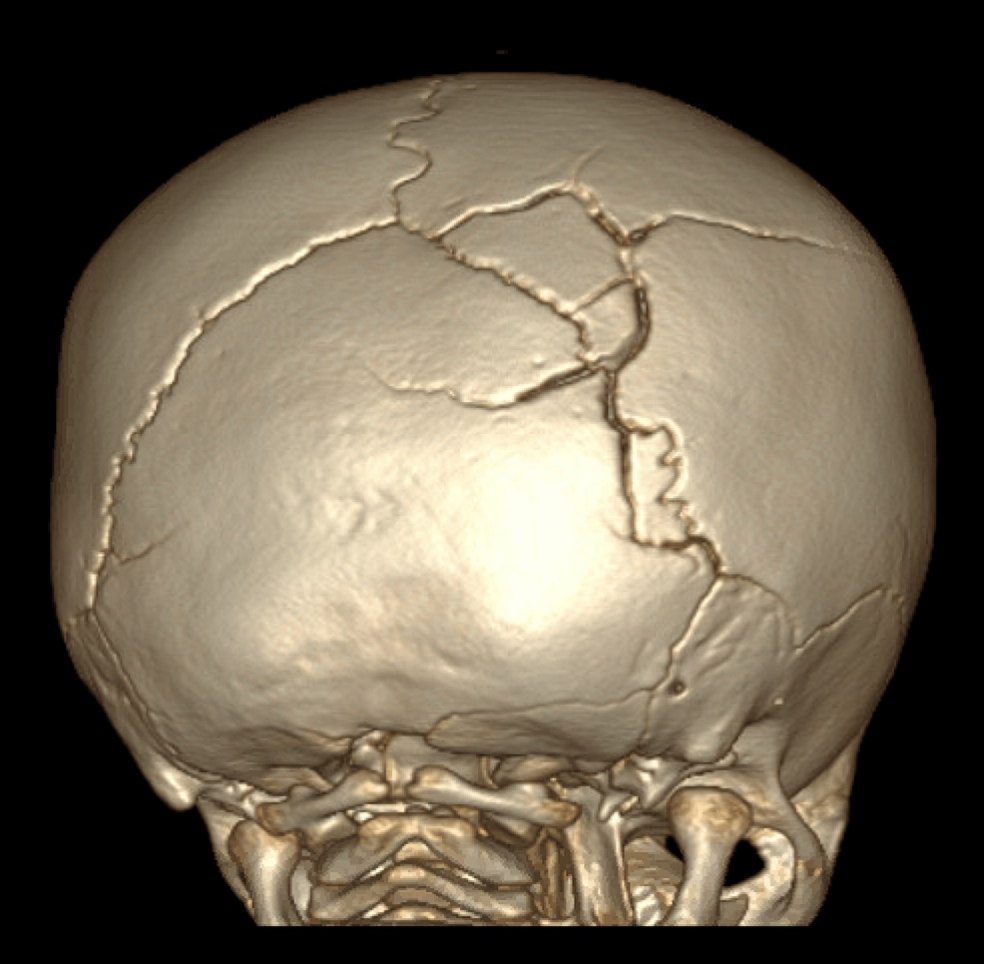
3D CT scan findings of a cranial fracture in a six-month-old female coronal view. The figure shows a fairly nondisplaced fracture of the right parietal and temporal bones, minimally extending into the right occipital bone; it is associated with overlying soft tissue swelling; it crosses suture lines and widens approaching the lambdoid suture line; no significant sclerotic edges are noted, signifying the recent manifestation requiring time for sclerosis.
